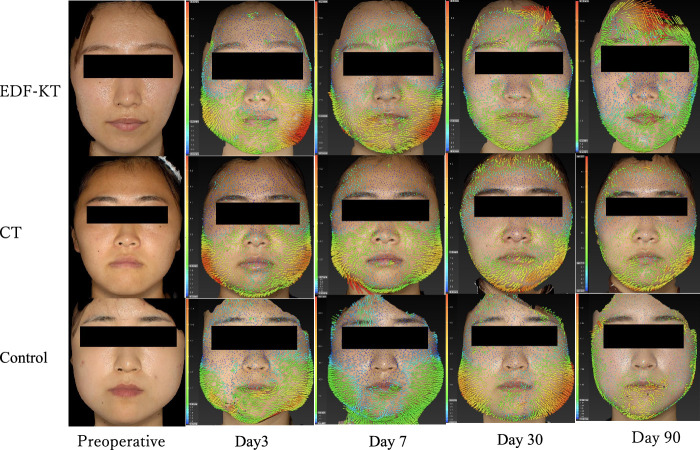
Superimposition and swelling measurement before surgery. CT, computed tomography; EDF-KT, epidermis, dermis, and fascia method-Kinesio taping.
